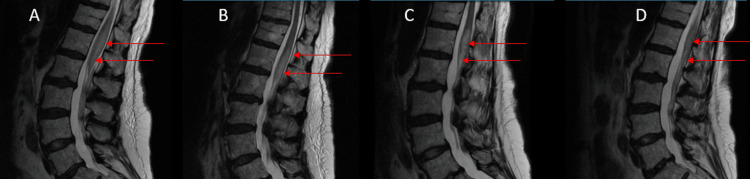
Non-contrast MRI of the lumbar spine. (A) At admission. (B) At discharge, nine days after admission. (C) Four months after discharge. (D) Six months after discharge. Serial images taken from presentation up to four months after admission show persistent lumbar spine signal changes. The MRI done in the sixth month shows improvement in the lesion after the discontinuation of abatacept.
